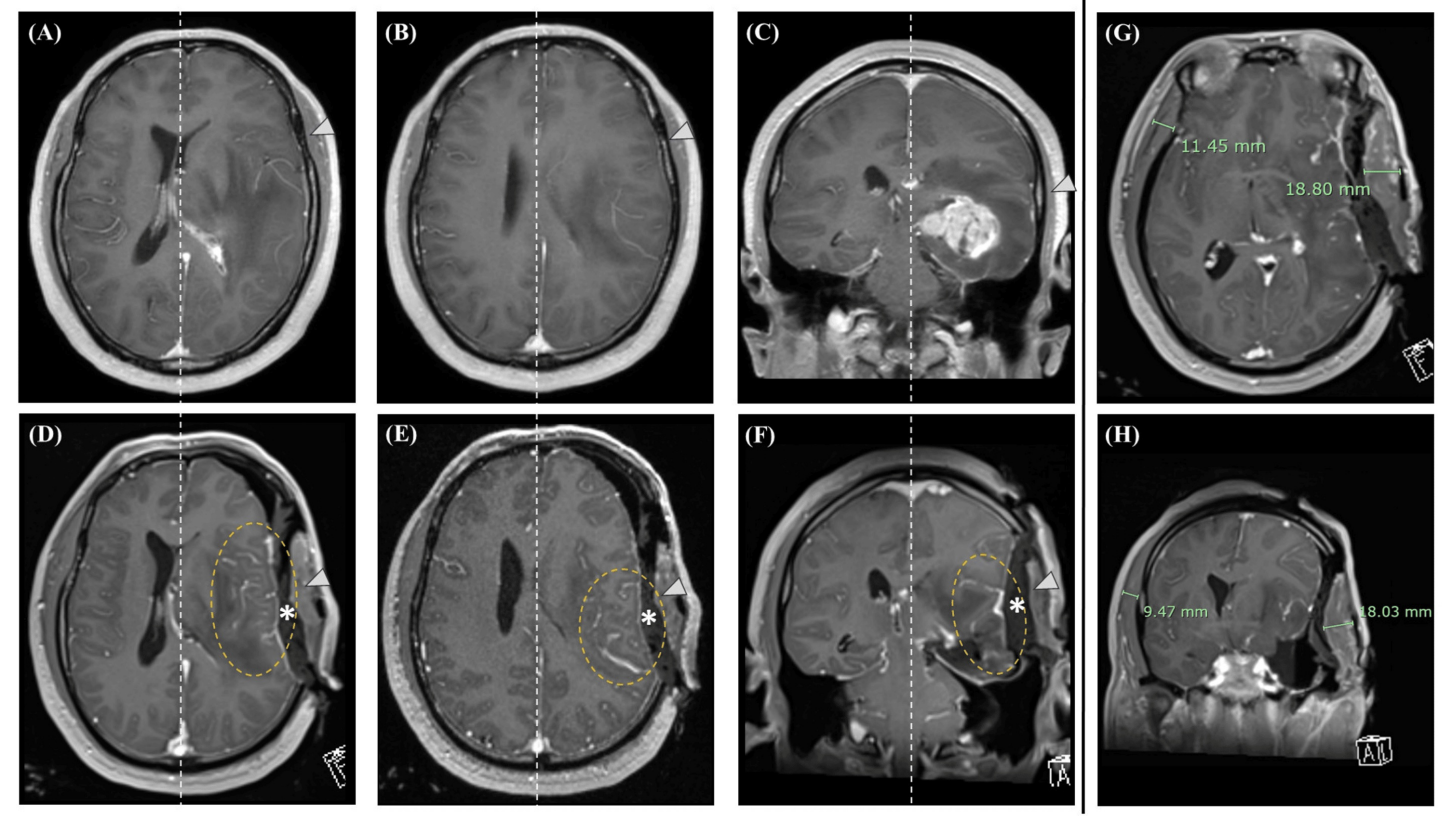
Comparison of pre- and intraoperative MRI findings Compared to the preoperative MRIs (upper column, A–C), intraoperative brain MRIs (lower column, D–F) demonstrated that the left cerebrum was compressed and deformed by the temporal muscle (arrowheads) and the inserted gauze (asterisks). The left cerebral veins were more dilated than those on the contralateral side (dotted circles). Vertical dotted lines indicate midlines. The maximum thicknesses of the temporal muscles on both sides are displayed in the axial (G) and coronal (H) sections. MRI: magnetic resonance imaging
